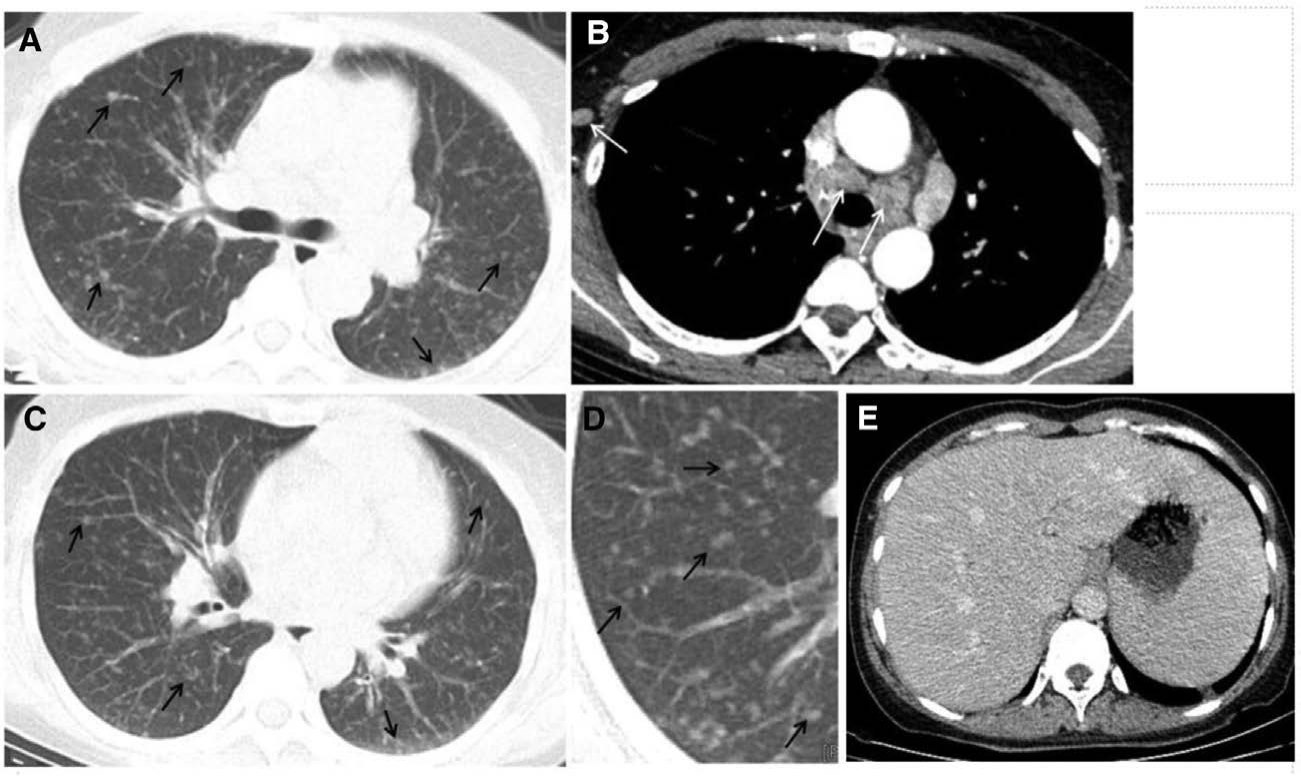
Axial contrast-enhanced chest CT scan: diffuse ground glass nodules in both lungs (A, C, D), multiple enlarged lymph nodes in mediastinum and axilla (B). Abdominal enhanced CT scan showed enlargement of the liver and spleen (E). CT = computed tomography.
